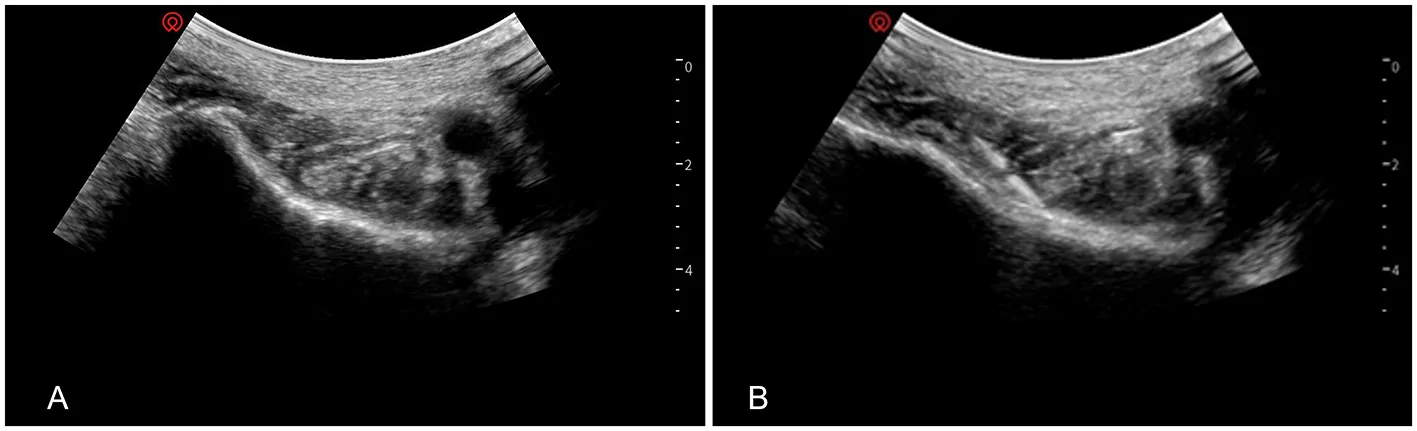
Ultrasound-guided pericapsular nerve group (PENG) block. (A) Pre-injection ultrasound image identifying the key anatomical landmarks. (B) Post-injection ultrasound image showing the spread deep to the psoas tendon over the superior pubic ramus.
